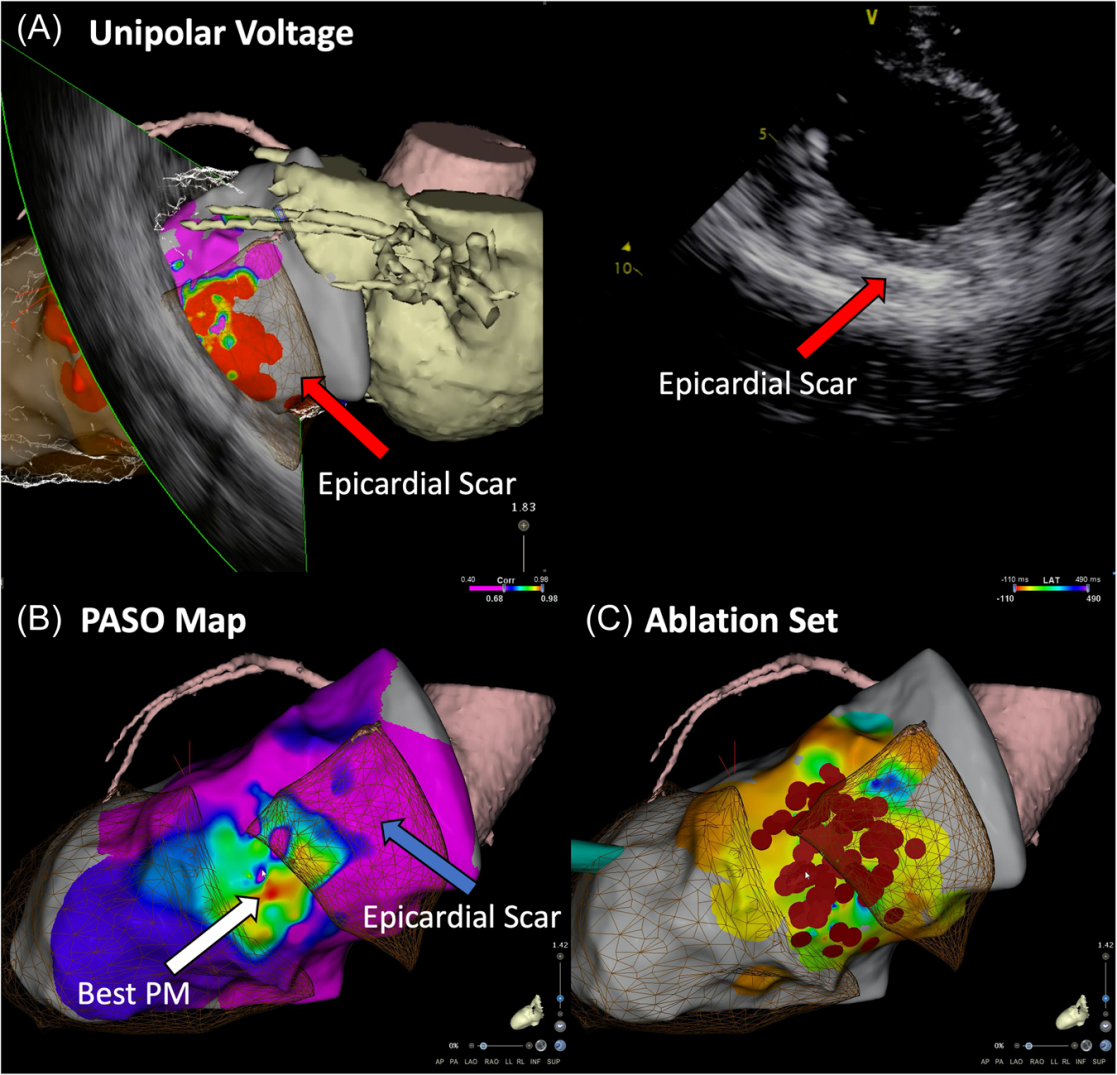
Ablation procedure. (A) ICE image of lateral scar (red arrow) with corresponding 3D CARTO map. (B) Map of pacing sites, demonstrating best match at border‐zone of basal scar (mesh area labeled with blue arrow). (C) Final ablation set over the mid‐basal lateral wall. ICE, intracardiac echocardiography
